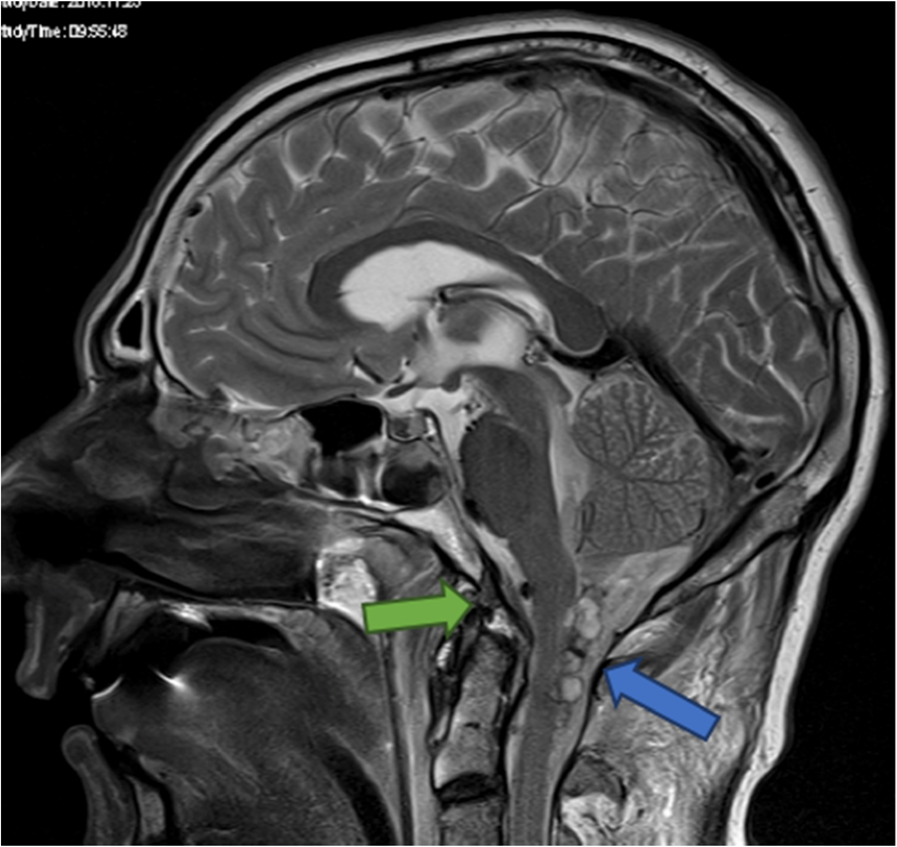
Magnetic resonance imaging scan of the brain and the upper cervical spine with atlanto-occipital dislocation showing cystic hemorrhagic lesions posterior to the spinal cord between C0 and C2 (*blue arrow*) and complete rupture of the apical odontoid ligament (*green arrow*)
